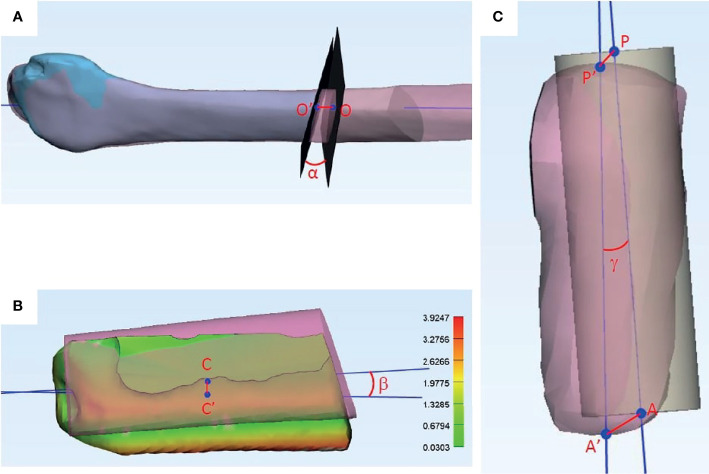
References used in accuracy analyses. (A) Fibula distal osteotomy accuracy analyses. Blue: Distal end of fibula after harvest. Pink: Fibula in preoperative virtual surgical planning. O: Center of the planned osteotomy plane. O’: Center of the actual osteotomy plane. O-O’: Axial deviation of the osteotomy plane. α: Angle deviation of the osteotomy plane. (B) Reconstruction segment accuracy analyses. Colored: Fibula segment at recipient site in postoperative CT scan. Pink: Fibula segment in preoperative virtual surgical planning. C: Center of the planned fibula segment. C’: Center of the actual fibula segment. C-C’: Center point deviation of the fibula segment. β: Angle deviation of the fibula segment. Absolute distance deviation represented in the color map. (C) Implant accuracy analyses. Yellow cylinder: Implant position in the virtual surgical plan. Pink cylinder: Actual implant position in the postoperative CT scan. P: Center point of implant platform in the virtual surgical plan. P’: Center point of implant platform in the actual implant. A: Apex of the implant in the virtual surgical plan. A’: Apex of actual implant placed. P-P’: Deviation in implant platform position (mm). A-A’: Deviation in implant apex position (mm). γ: Angle deviation of long axes of implants.
